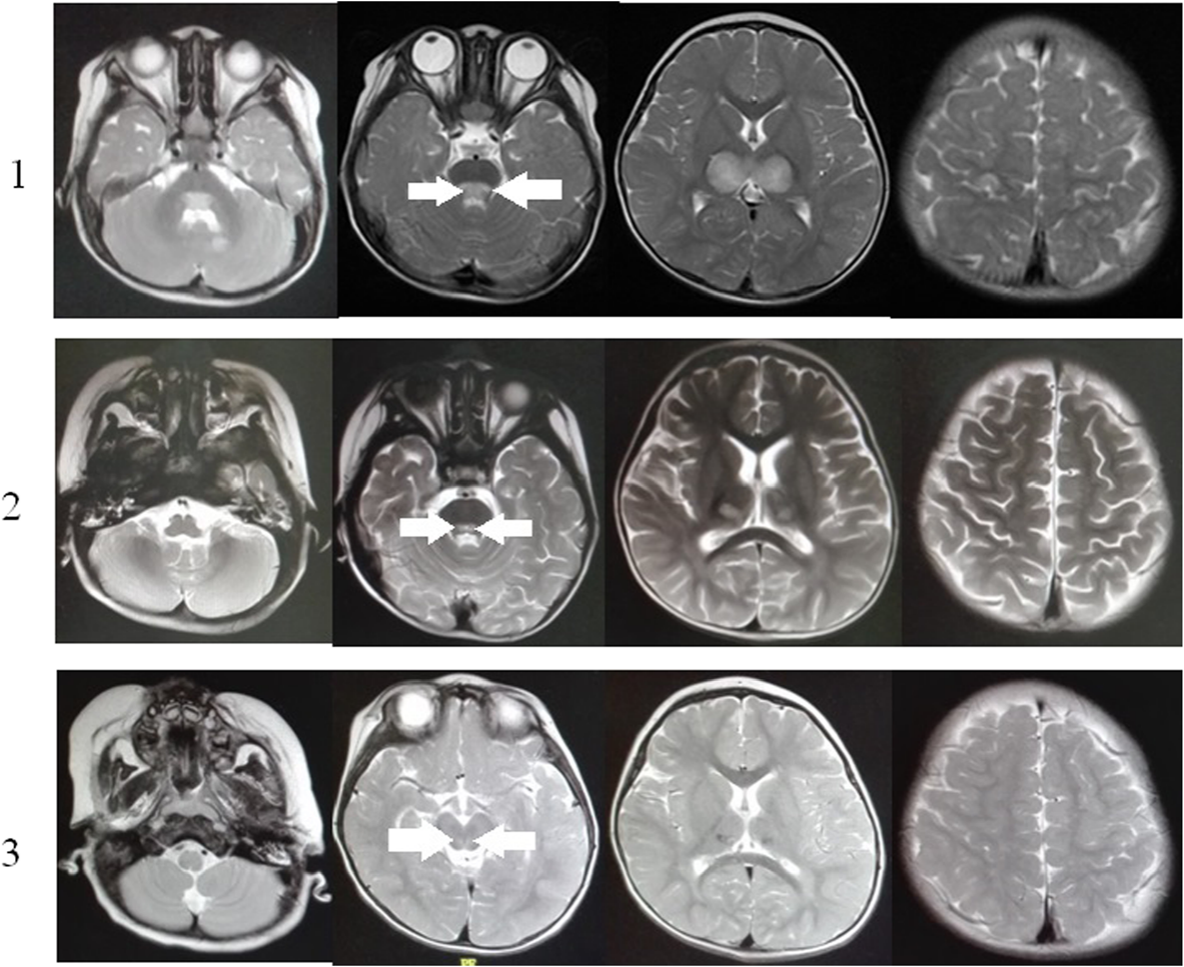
The brain T2-weighted MRI images. The leisions in the dorsal part of pons (*arrows*) were considered to cause the bilateral abducens palsy. *1*: T2-weighted MRI image showing areas of hyperintensity involving the *left* cerebellar hemisphere, the dorsal part of pons, thalamus, and *right* frontal lobe. *2*: T2-weighted MRI image after 15 days showing areas of hyperintensity that still involved the dorsal part of pons and thalamus, but not the *left* cerebellar hemisphere and *right* frontal lobe. *3*: T2-weighted MRI image after 6 months showing areas of hypointensity involving the dorsal part of pons and thalamus
